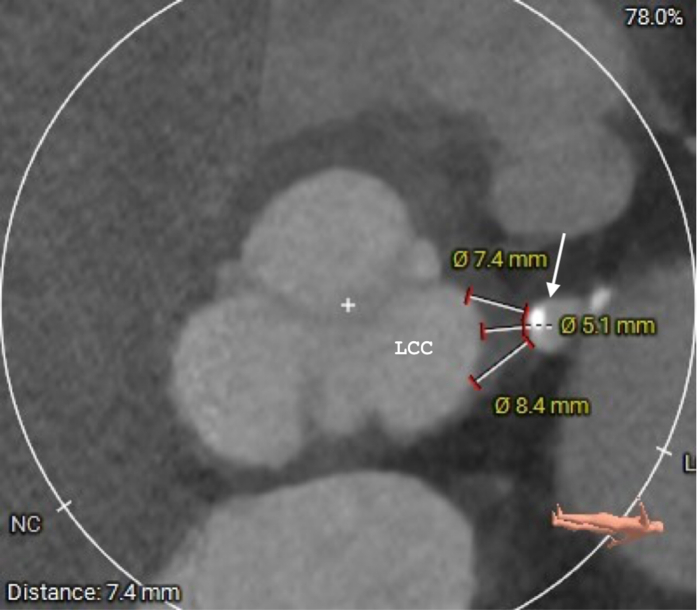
Computed Tomography Showing Distances From Left Main Stump (Arrow) to Aortic Root LCC = left coronary cusp; NC = noncoronary.
